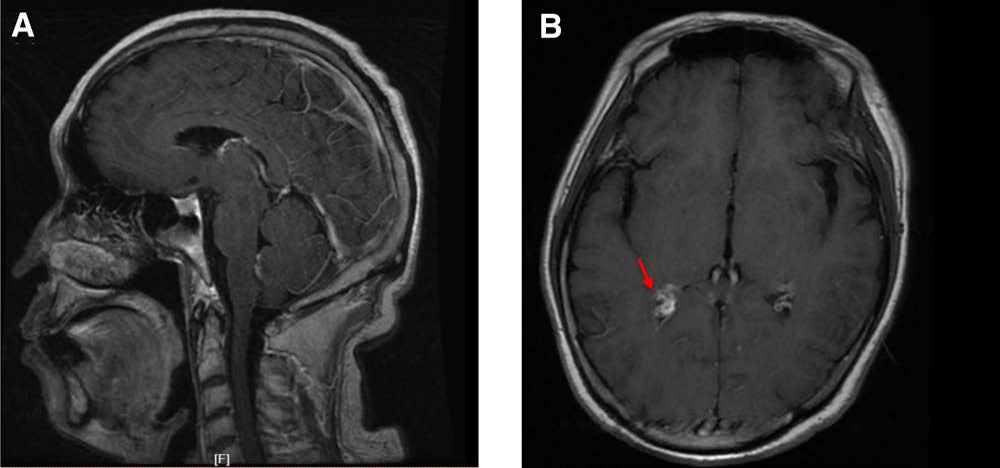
Brain magnetic resonance image (T1-weighted sequence with gadolinium). The sagittal sequence shows the leptomeningeal of the bilateral cerebral hemisphere, cerebellar hemisphere, and brain stem meninges were slightly thickened and enhanced (A). From the axial sequence, the right trigone of the lateral ventricle showed a gadolinium-enhanced lesion (B).
